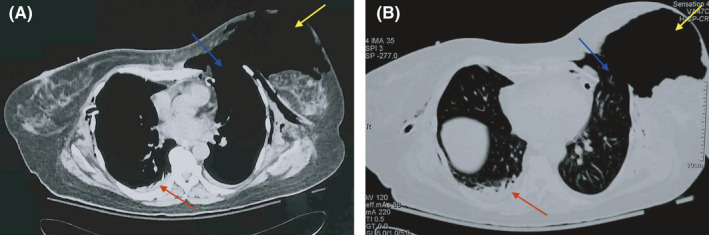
CT scan images of the patient's chest showing large left parasternal lung herniation (yellow arrow), costochondral disruptions (blue arrow), and a small left hemothorax (red arrow)
